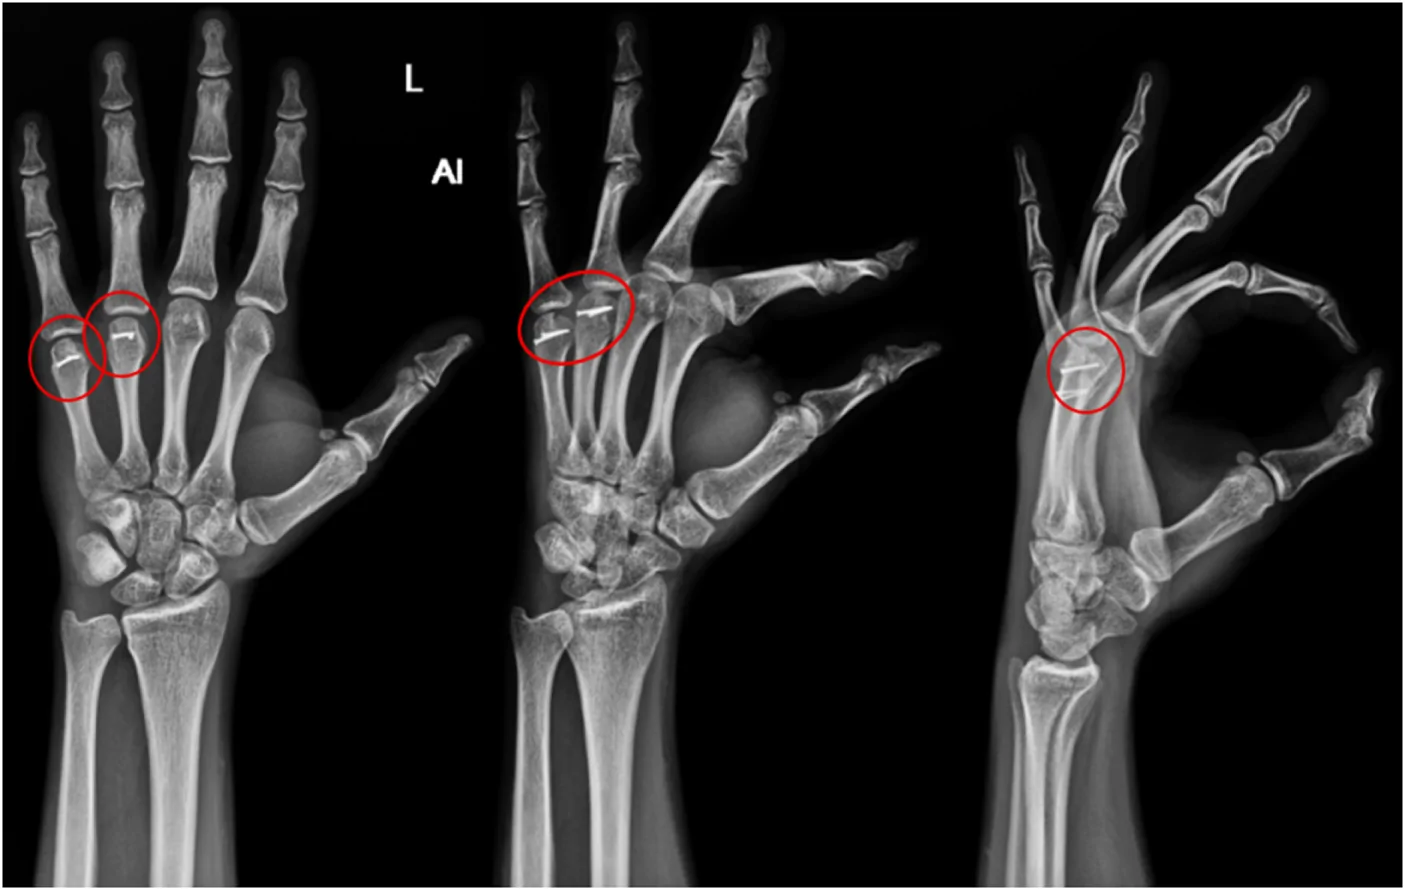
Posteroanterior, oblique, and lateral radiographs of the left-hand showing reduction of the fourth and fifth metacarpal fractures with good bony alignment at 2 months post-op.
